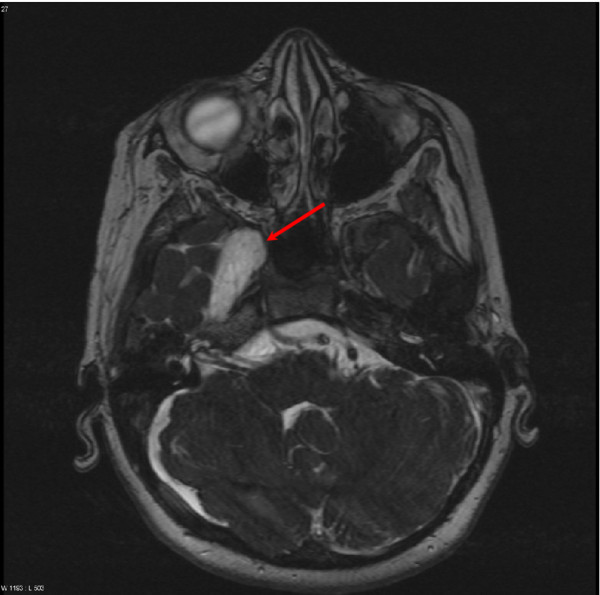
Arachnoidal cysts. MRI Scan of the brain. Coronar, T2-weighted. Cerebellar dysplasia is seen on the right side. The arrow indicates a large arachnoidal cyst.
